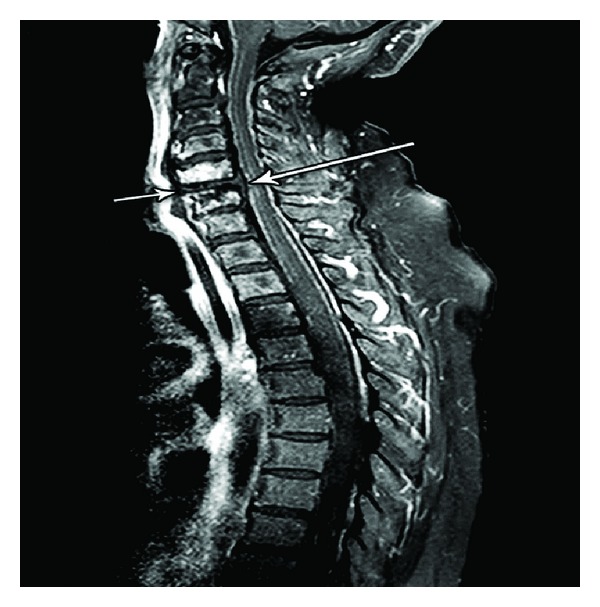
T1 MRI with fat suppression after IV Gadolinium in sagittal plane, demonstrating prevertebral collection (short arrow), with extension through the disk space into the epidural space (long arrow).
